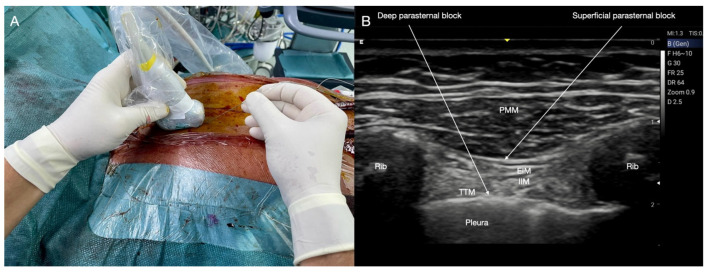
Superficial and deep Pecto-Intercostal Plane block. (A) Patient, probe, and needle position. (B) Superficial and deep parasternal block ultrasound landmarks. PMM: pectoralis major muscle; EIM: external intercostal muscle; IIM: internal intercostal muscle; TTM: transversus thoracis muscle; Rib: rib; Pleura: pleura.
